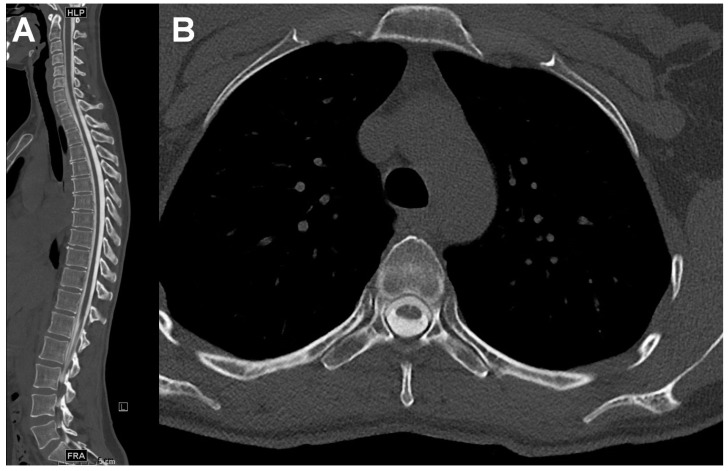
Image examples of a CT myelography of the whole spine of a 36-year-old female patient with suspected cerebrospinal fluid (CSF) leak in sagittal (A) and axial (B) reconstruction at level Th4.
